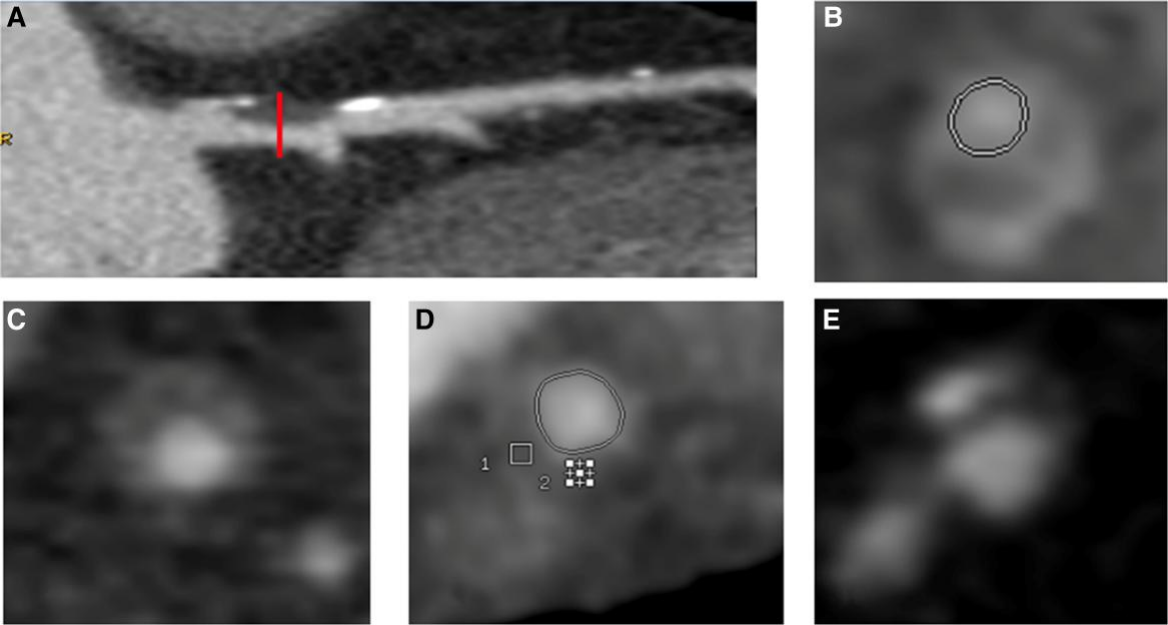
Computed tomography coronary angiography (CTCA) images of four ‘high-risk’ plaque phenotypes. (*A*) Positive remodelling: ratio of the outer vessel diameter of the plaque divided by outer diameter of the vessel, proximal and distal, >1.1. (*B*) Napkin ring sign: low-attenuation area adjacent to the coronary lumen, surrounded by a ring of higher attenuation (<130 HU). (*C* and *D*) Low attenuation: non-calcified plaque with internal attenuation < 30 HU.^8^ (*E*) Spotty calcification: plaque with punctate calcium.
